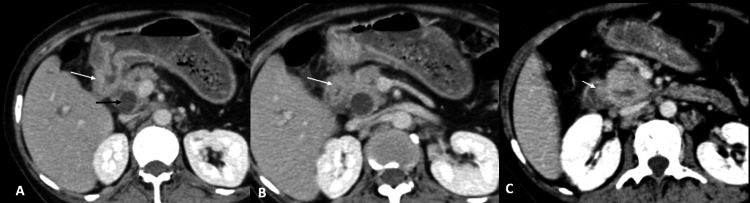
Gastric adenocarcinoma Axial sections of the CECT of the abdomen show mucosal thickening involving the antrum, duodenum, and ampulla (white arrows in Figure 1A, Figure 1B, and Figure 1C, respectively) with loss of mural stratification. CECT: contrast-enhanced computed tomography
